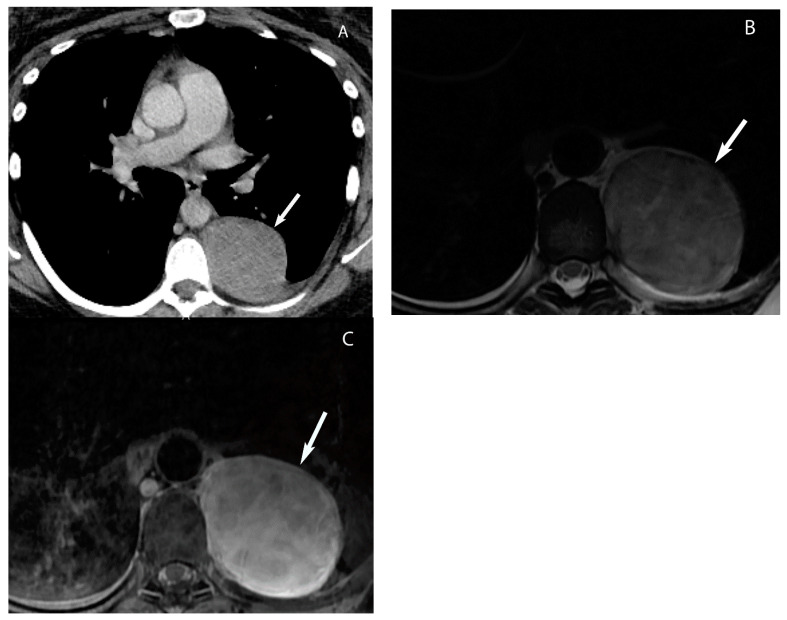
Schwannoma. CT (A) shows a well-defined spherical shaped mass in the left paraspinal region (arrow). MRI (B,C) shows the mass is hyperintense upon T2W imaging (arrow in (B)) and enhances with gadolinium (arrow in (C)).
